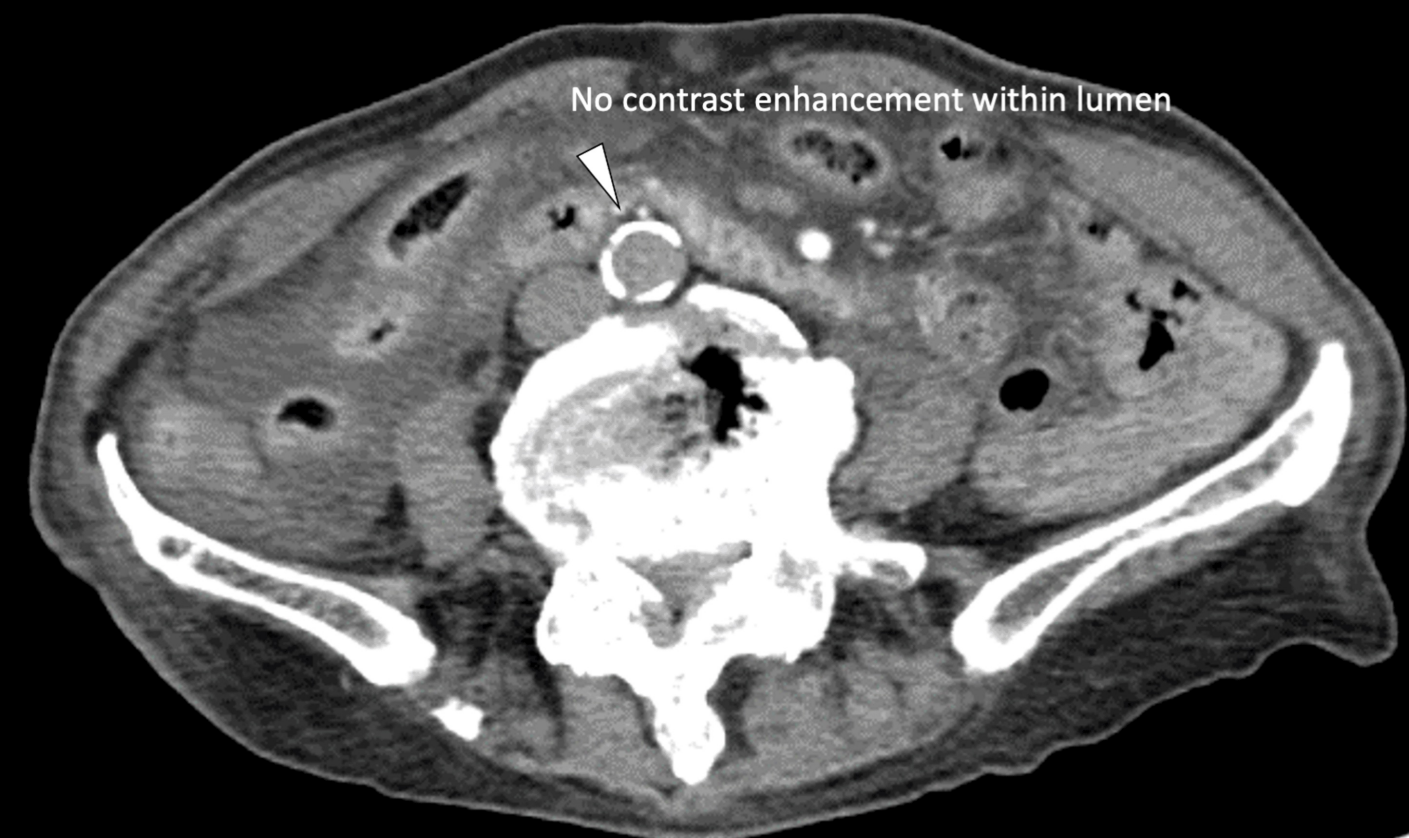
Axial arterial phase CT showing complete distal abdominal aortic occlusion. Axial contrast-enhanced CT image in the arterial phase on day four of hospitalization showing complete thrombotic occlusion of the distal abdominal aorta (arrowhead). The absence of contrast enhancement within the aortic lumen confirms a lack of arterial flow. This finding supports the diagnosis of acute abdominal aortic thrombosis and corresponds with the patient’s acute bilateral lower limb ischemia.
